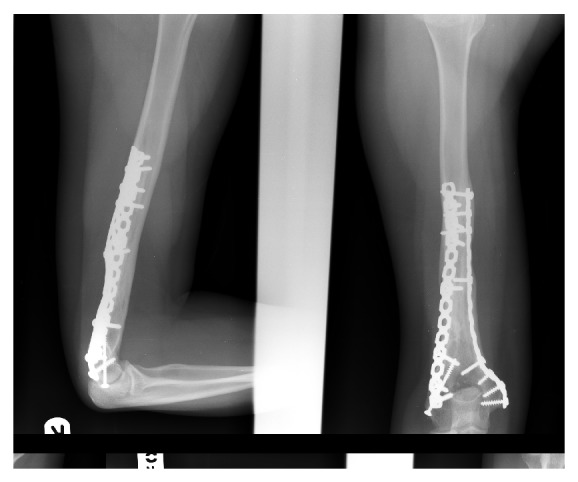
Lateral and anteroposterior X-ray 10 years after surgery. These show full healing and consolidation of the graft with excellent union at both ends of the graft.
